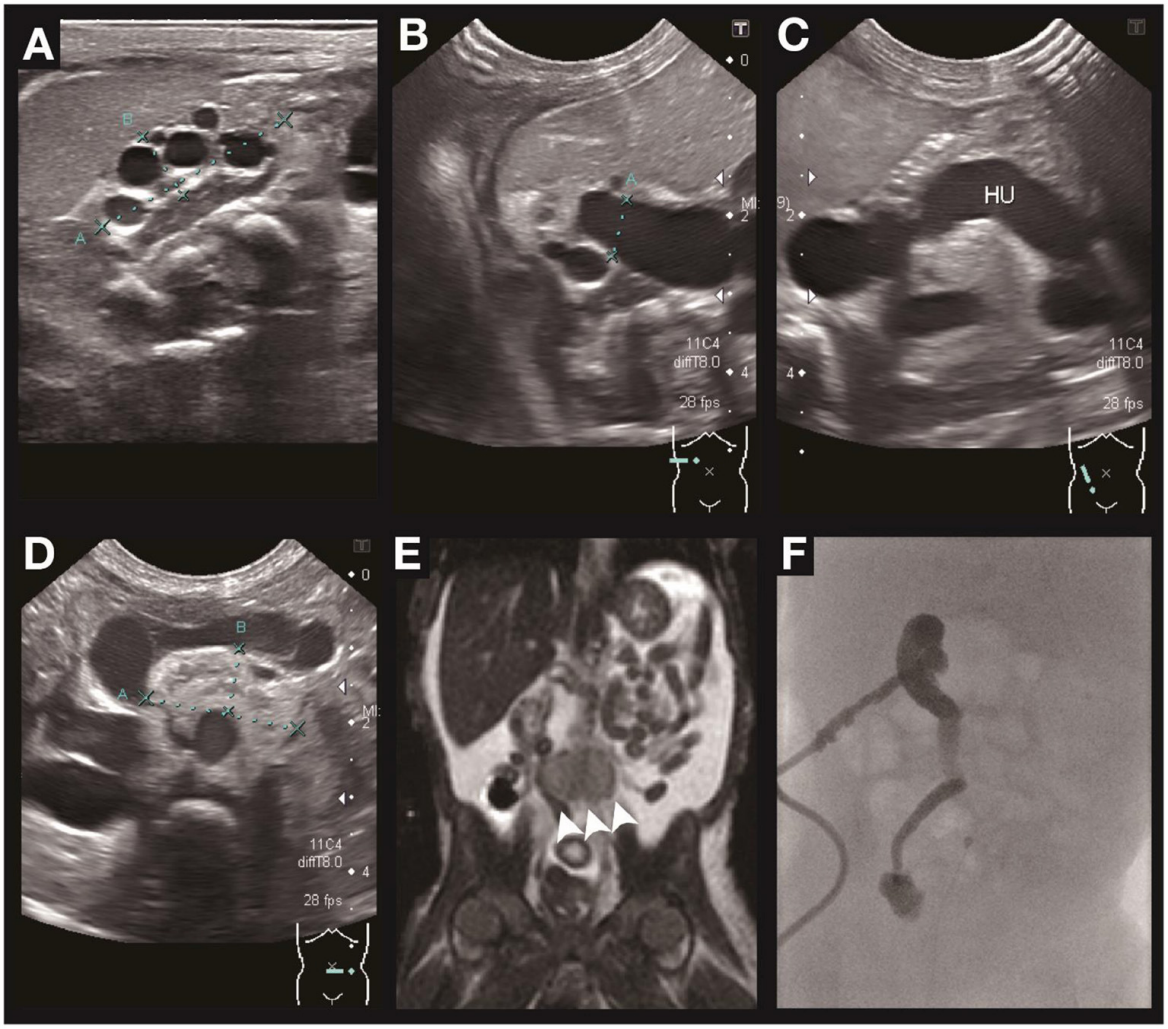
Renal ultrasound, MRI, and antegrade pyelography of case B. Ultrasonography of the urinary systems depicts small size of the right kidney with dysplasia and multiple cysts formation (A), right hydronephrosis (B), right hydroureter (C), and left ectopic kidney with dysplasia and hypoplasia. (D) MRI of the abdomen (E) confirmed the ectopic left kidney anterior to the aortic bifurcation (arrowhead). Antegrade pyelography (F) depicts high grade obstruction at the distal third of the right ureter with contrast medium not passing into the urinary bladder.
